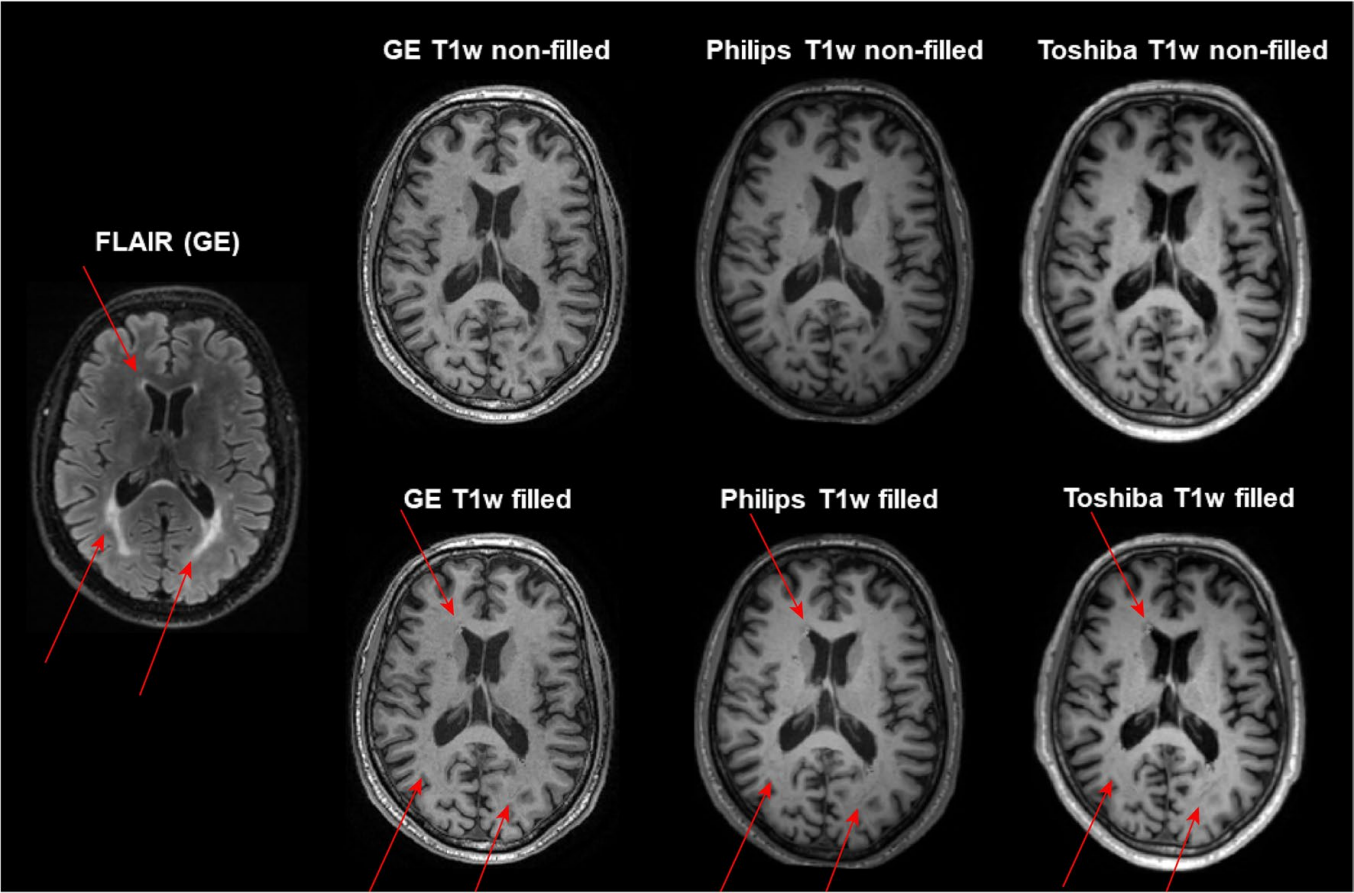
Example of non-filled and filled T1w images and the additional FLAIR scan of the same subject. Red arrows indicate the lesions on FLAIR and the filled lesions on the T1w images
